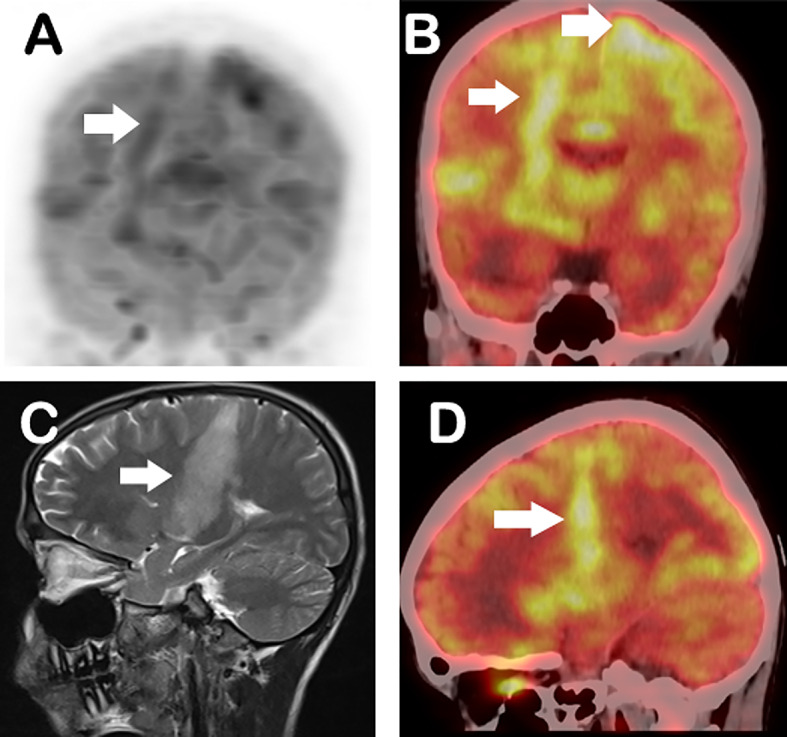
17-year-old boy with brain SOL (A) ^18^F-FDG PET/CT MIP (Maximum intensity projection), (B, D) sagittal and coronal fused PET/CT images show minimally FDG avid (SUVmax 7.1) ill-defined linear hyperdense areas involving right frontal lobe, right basal ganglia, and right thalamus extending till right cerebral peduncle of midbrain, with perilesional edema (arrows A, B, D). Another Focal FDG avid subtle hyper density is noted in the left high frontal cortex, SUVmax 9.1 (arrows in B). (C) MRI sagittal view demonstrated T2 hyperintensity along the corticospinal tract from the cortex to the subcortex, tracking along the white matter tract to the midbrain, a finding termed as “Tunnel Sign” (arrow in C)
